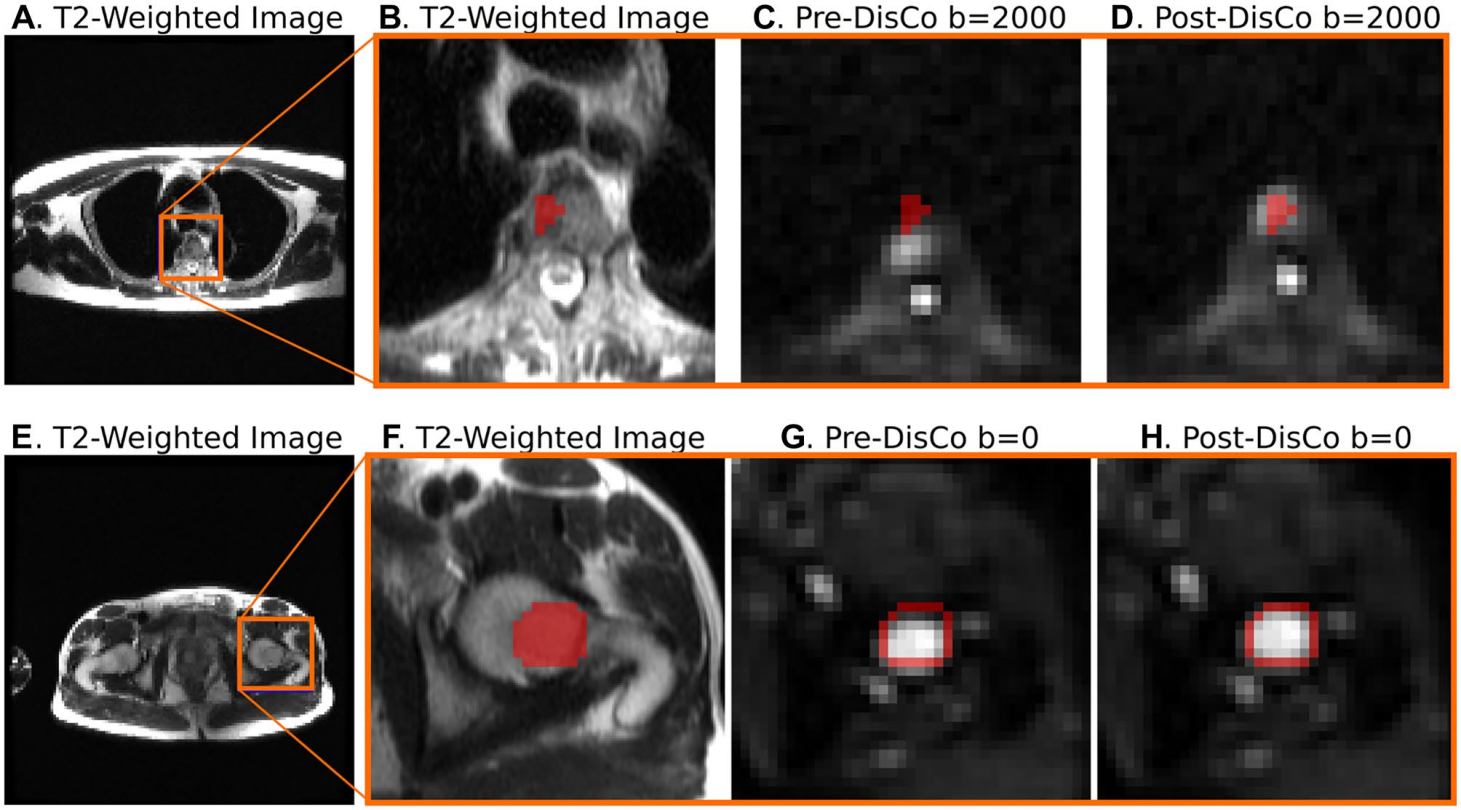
Examples of bone metastases as seen on b = 0 s/mm^2^ DWI pre-and post-DisCo. The top row (A–D) shows a vertebral lesion in the Thorax Station. The bottom row (E–H) shows a lesion within the left femoral head in the Pelvis Station. (A,E) T2-weighted images at the corresponding level with an orange bounding box drawn around the lesion. (B,F) T2-weighted images zoomed in to visualize lesion and surrounding area. (C,G) Zoomed in DWI b = 2000 s/mm^2^ and b = 0 s/mm^2^ images before DisCo, respectively. (D,H) Zoomed in DWI b = 2000 s/mm^2^ and b = 0 s/mm^2^ images after DisCo, respectively. Outlines of the lesion annotations are overlaid in red. In both examples, the lesions were translated anteriorly following DisCo. Without DisCo, lesion localization may have erred posteriorly. *DisCo* distortion correction.
